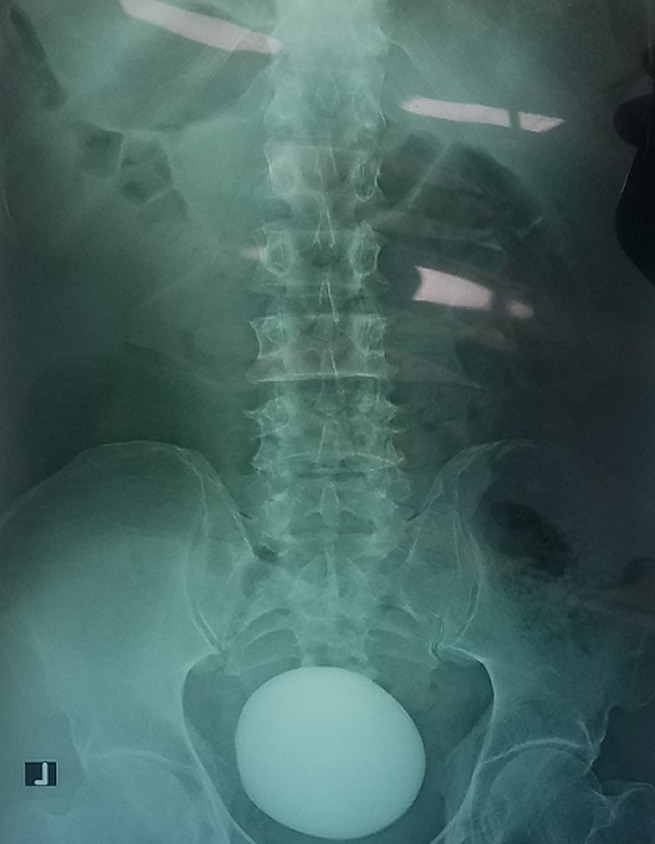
giant bladder stone in plain radiography
